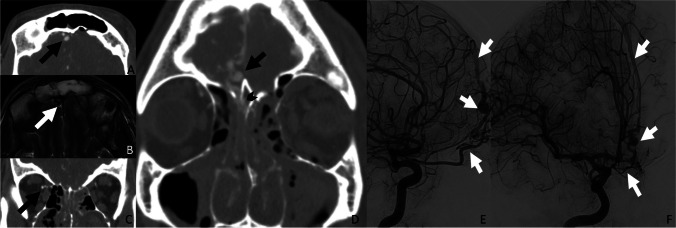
ACF dAVF. A 45-year-old male presented with severe craniofacial trauma involving the frontobasal distribution with skull base fractures extending along the ethmoid plates and left sphenoid sinus wall. The initial CTA demonstrated enlarged cortical veins along the right anterior cranial fossa (A) and prominent right ophthalmic and ethmoidal arteries (C). The MRI showed prominent flow voids of the enlarged cortical veins (B). On the CTA, the fistula point (black arrowhead) is centered at the fractured cribriform plate (D). Lateral and oblique internal carotid artery injections on the initial DSA demonstrated a fistulous connection between the ethmoidal branches of the ophthalmic artery with early venous drainage into the superior sagittal sinus (E and F)
